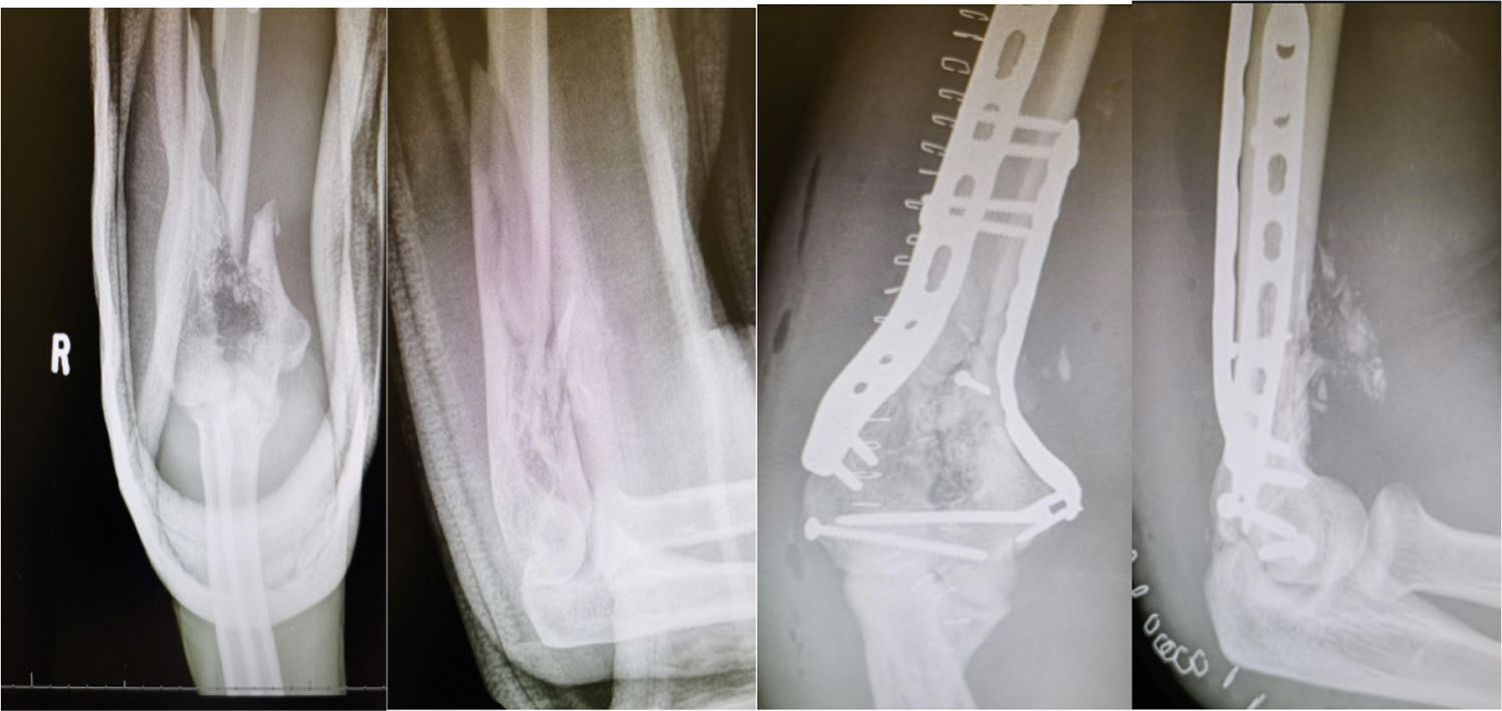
Pre-operative and post-operative radiographs of a gunshot distal humerus fracture treated with open reduction and internal fixation
